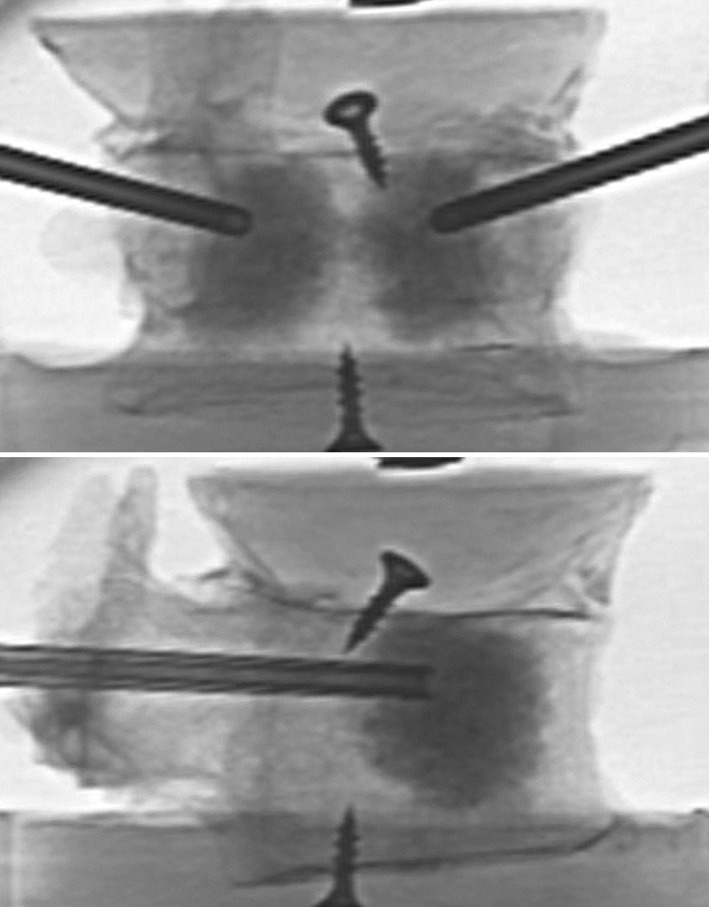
Radiographs in anteroposterior and lateral projections after augmentation of the vertebral body with the triphasic bone implant material AGN1
